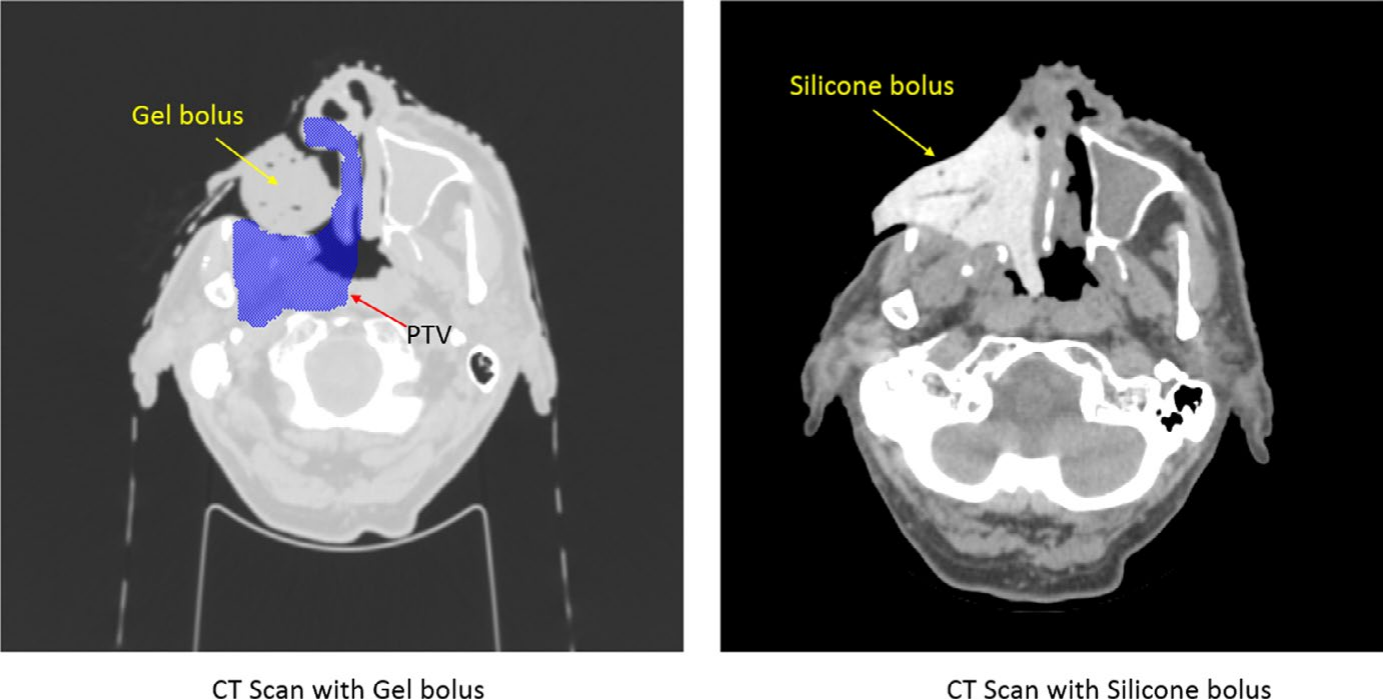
Comparison of the CT scans of the patient using different bolus materials. The left panel uses liquid gel with food wrap film; the right panel uses silicone. As shown in the left panel, the target includes the inner surface of the cavity
